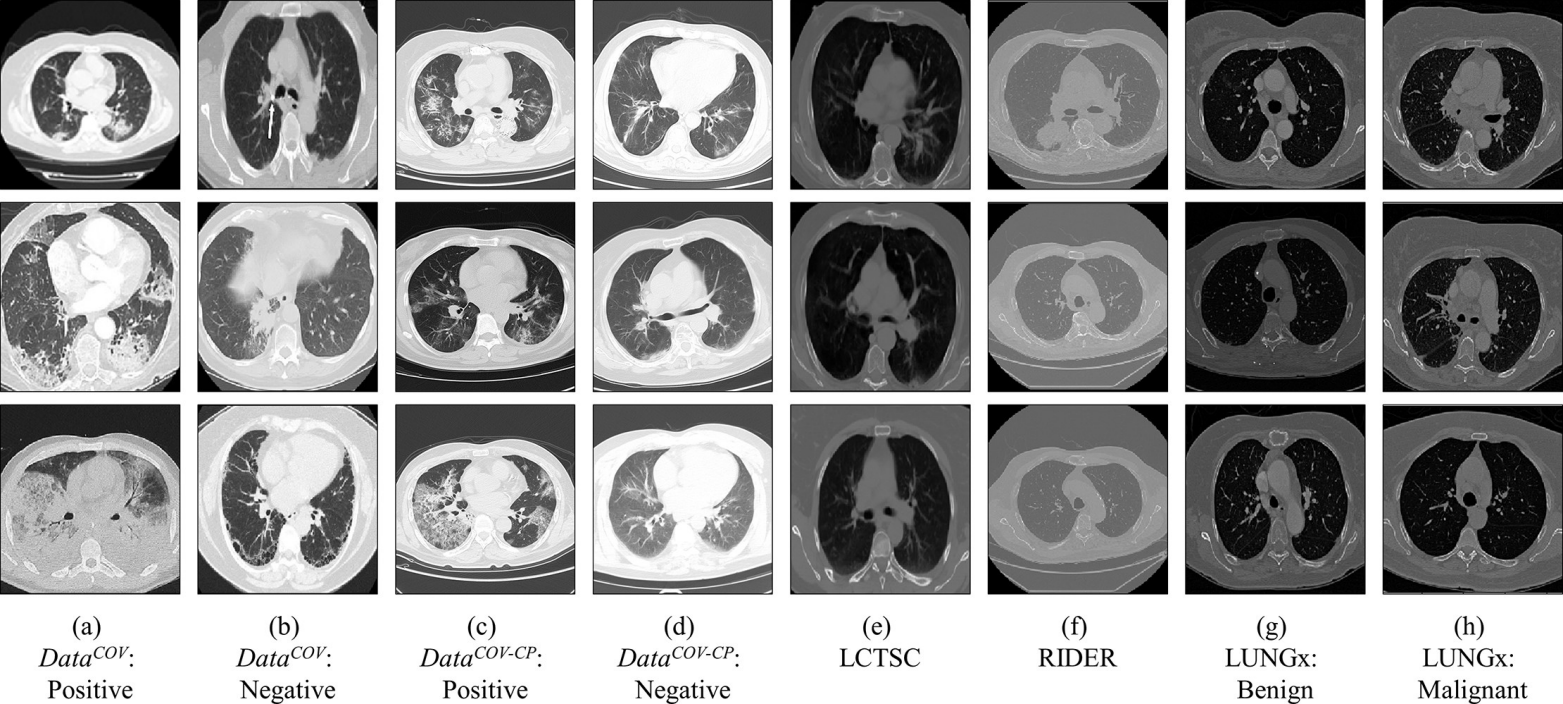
Exemplary CT images of the COVID-19 and public datasets.
